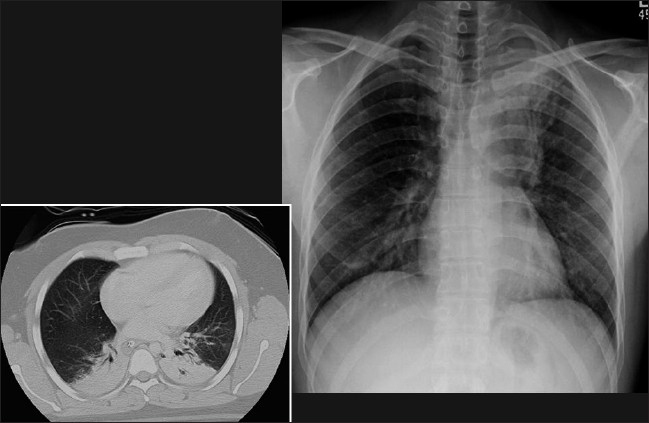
A chest radiograph (right) showing consolidation of left upper zone associated with an air bronchogram secondary to hospital-acquired pneumonia. The left image is an axial CT scan depicting an air bronchogram with bilateral pneumonic consolidation in another patient
